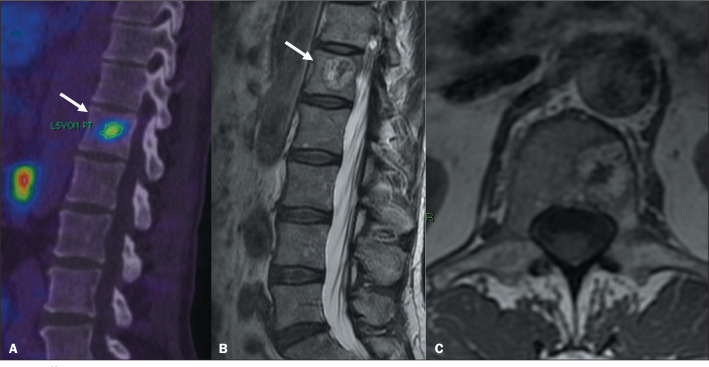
^68^Ga-PSMA PET/CT of a 67-year-old patient with biochemical
